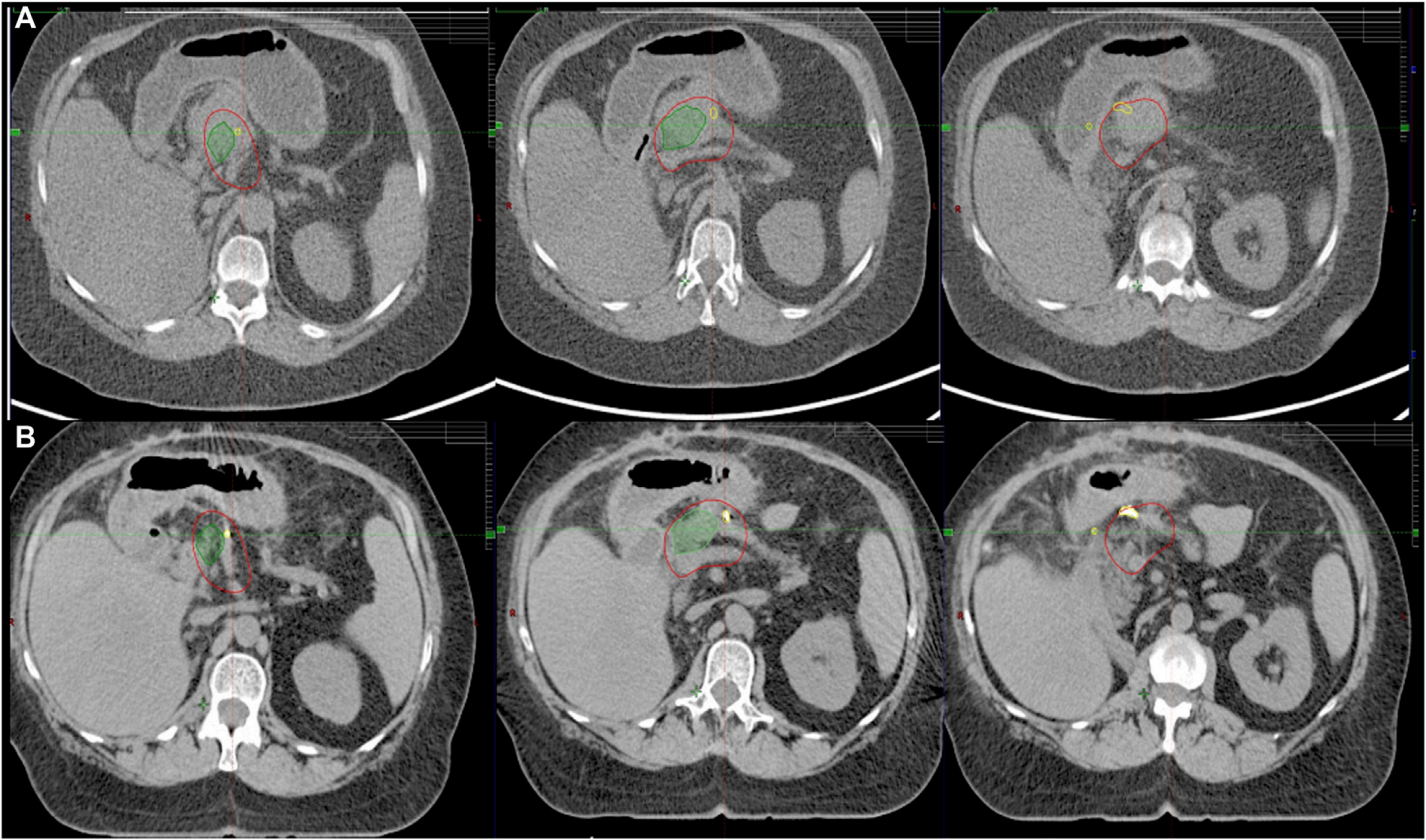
Corresponding axial CT slices on diagnostic scan (A) and treatment planning CT (B) fused by rigid registration. LAPC with abutment of gastric wall. Residual tumor (green), CTV (red). Note the implanted titanium clips (yellow) between the target volume and OARs assisting in target volume delineation.
